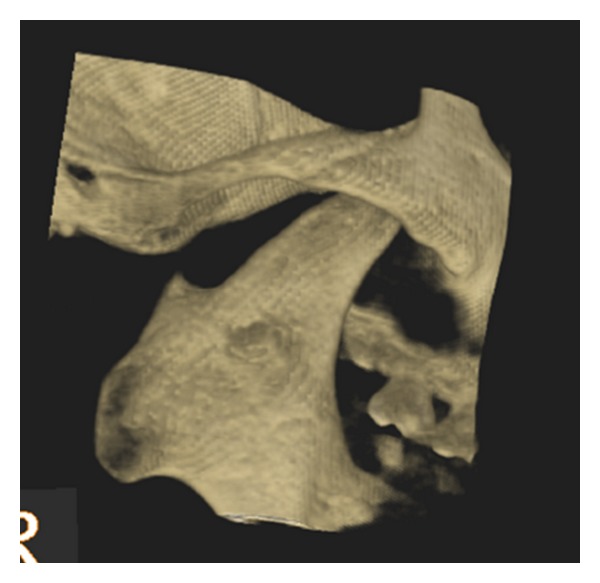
3D CBCT right oblique lateral shows absence of glenoid fossa and complete absence of the condyle.
